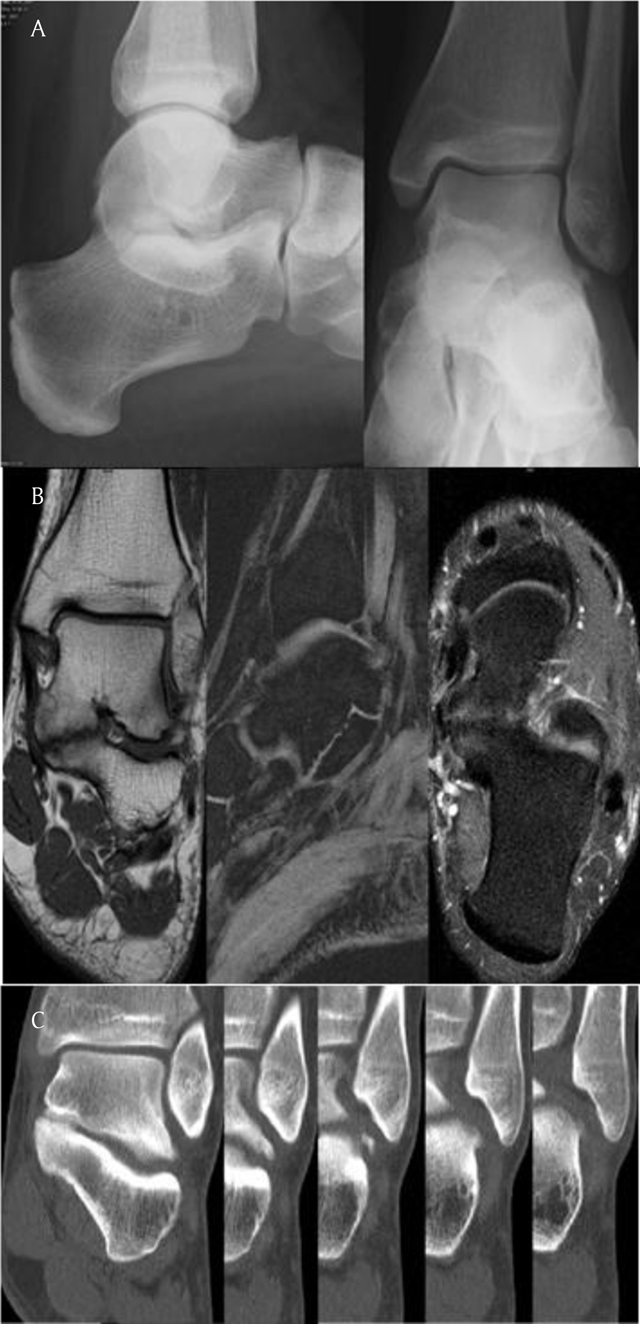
One year later, the same patient complains about newly arisen pain in the left hindfoot. (a) In the lateral view there is an ‘incomplete C-sign’, the sustentaculum is almost in perfect ‘brick’ shape. (b) MRI shows another coalition of the middle talocalcaneal facet. The MRI signal characteristics are in favor of a strong cartilaginous and less fibrous components and two subtle almost complete bony bridges across the joint space. Note that the orientation of the facet is less angulated out of its normal anatomic plane than in the right foot but there is more bone marrow edema pattern around the joint. (c) In this same patient’s left hindfoot, CT makes us appreciate an additional small bony bridge at the lateral aspect of the posterior talocalcaneal joint facet, consistent with an ‘extraarticular coalition’. This is a finding that should not be missed if surgery is considered.
